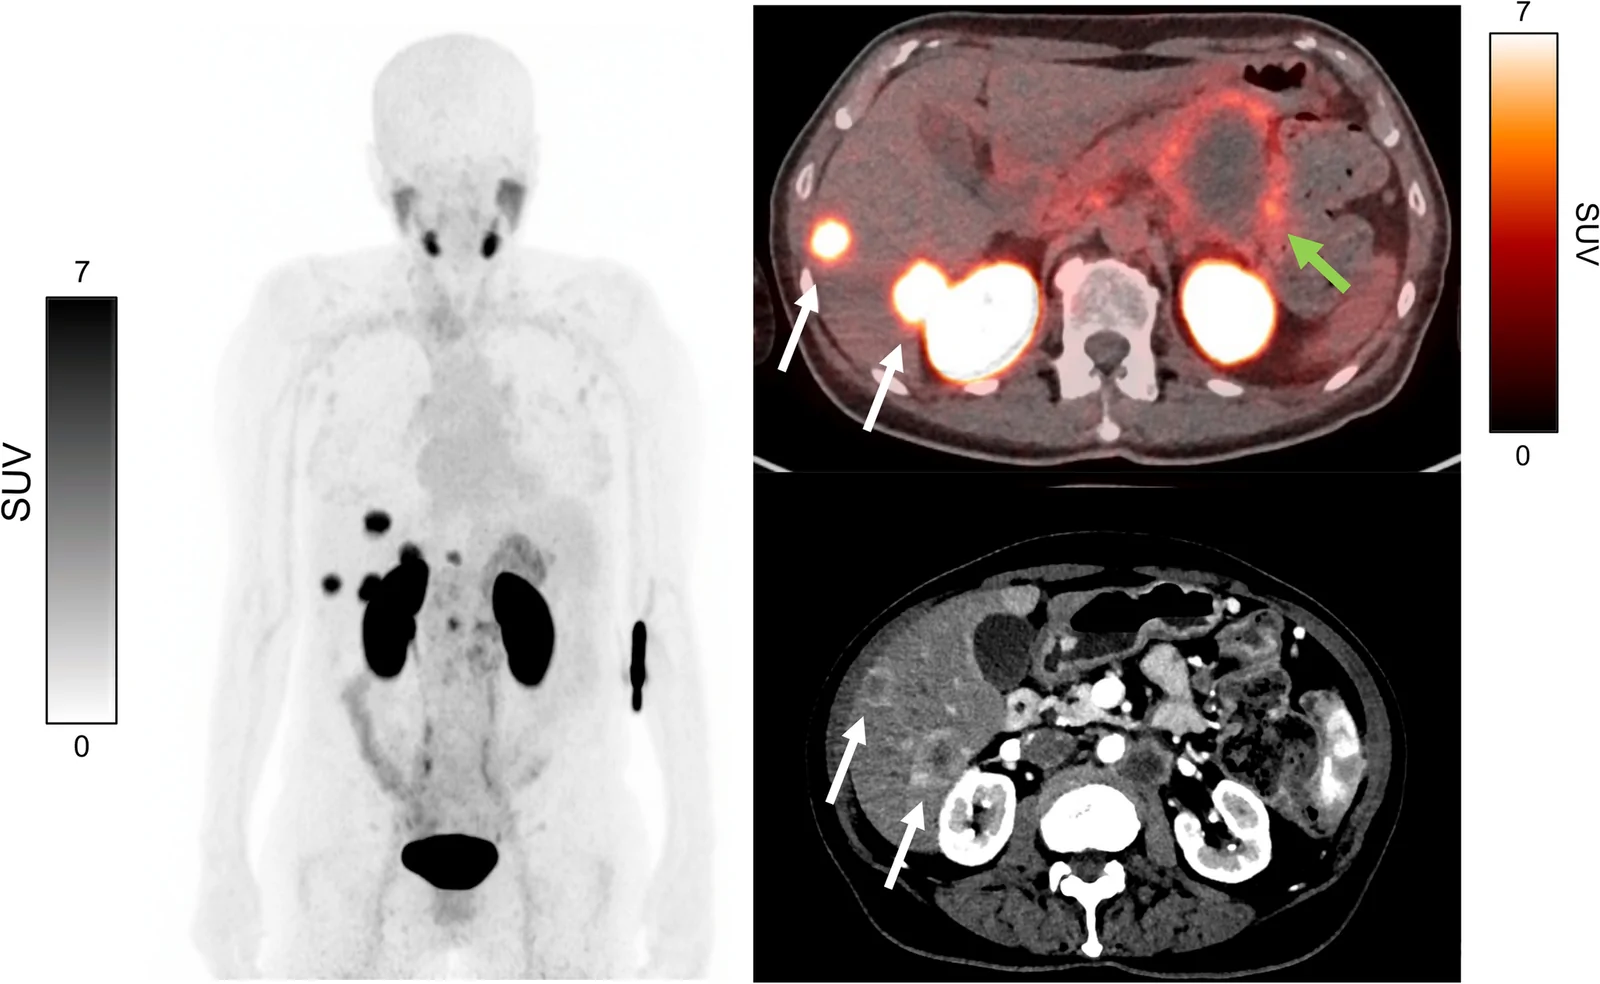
Comparison of standardized uptake values (SUV) between primary, different kinds of metastasis, physiologic uptake in the liver and blood pool. The maximum SUV (SUVmax) is shown in green, and the mean SUV (SUVmean) is shown in black
